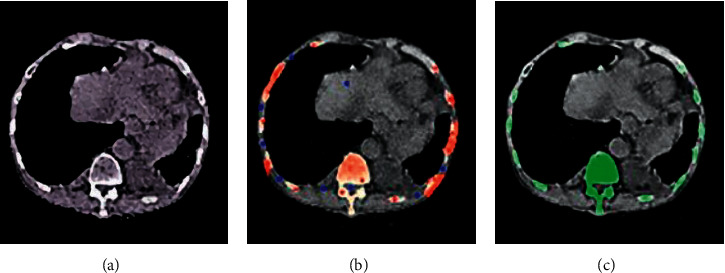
Comparison of CT image effects under algorithm reconstruction.
